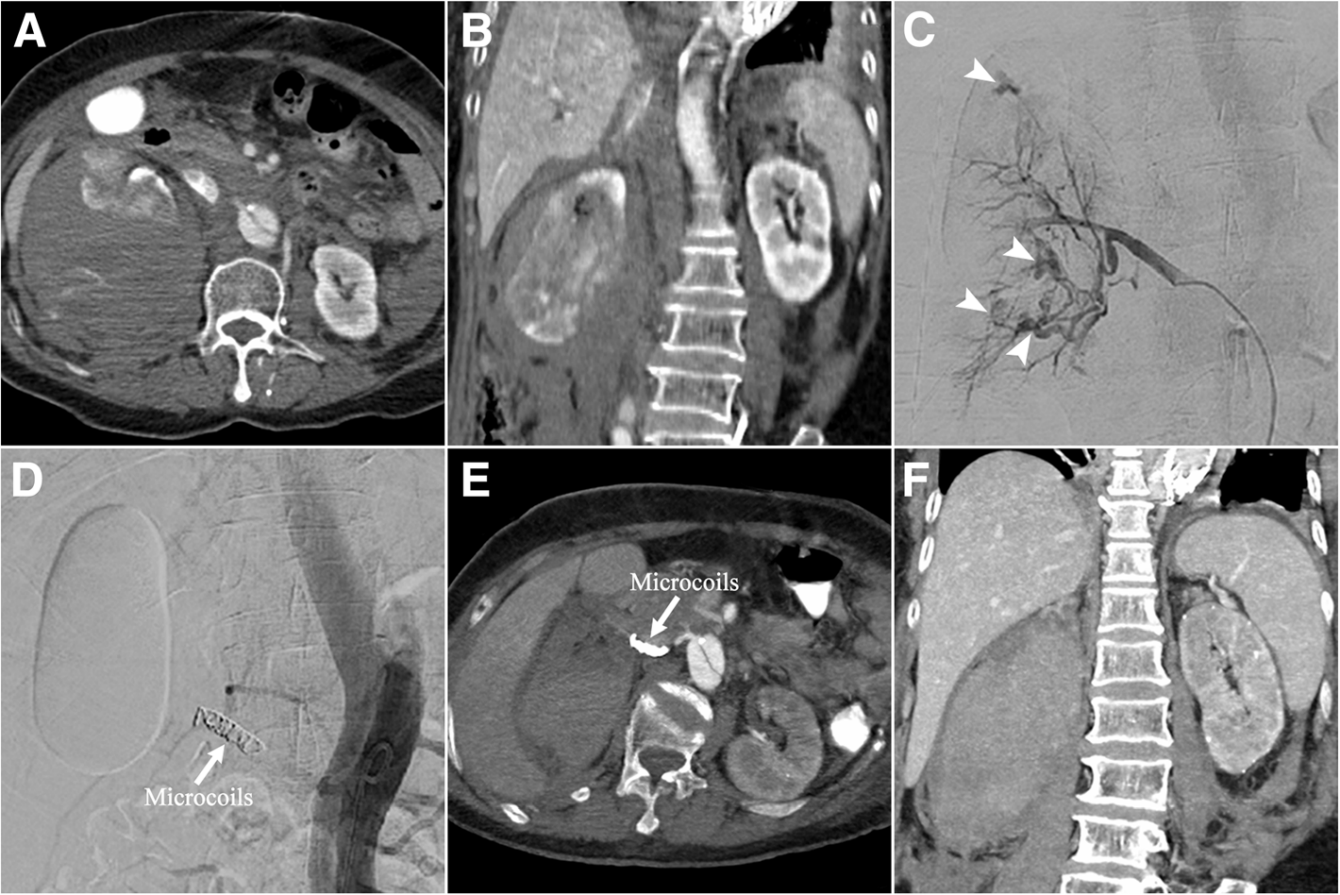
(a) Transverse and (b) coronal computed tomography angiography showed a massive right perinephric hematoma. (c) Right renal angiography detected multiple tortuous vascular branches with diffuse perinephric bleeding (arrowheads), and (d) the main trunk of right renal artery was embolized with microcoils. (e, f) Pre-discharge computed tomography angiography showed no signs of hemorrhage of the right kidney
